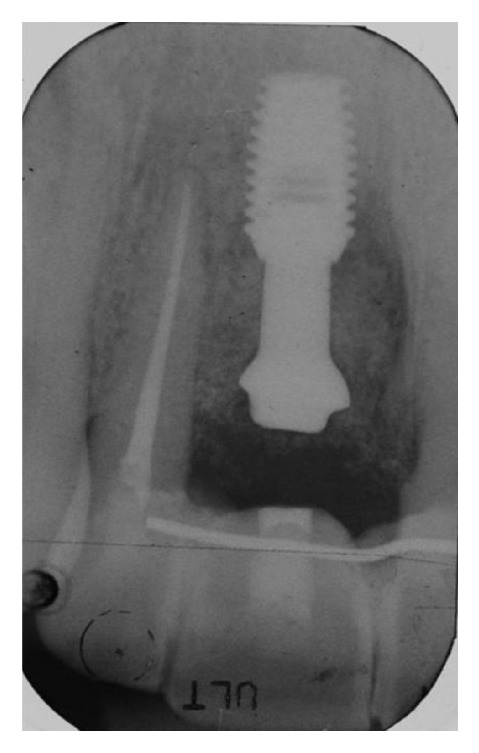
Radiograph at four months of healing showing good integration of the implant and the grafted material.
